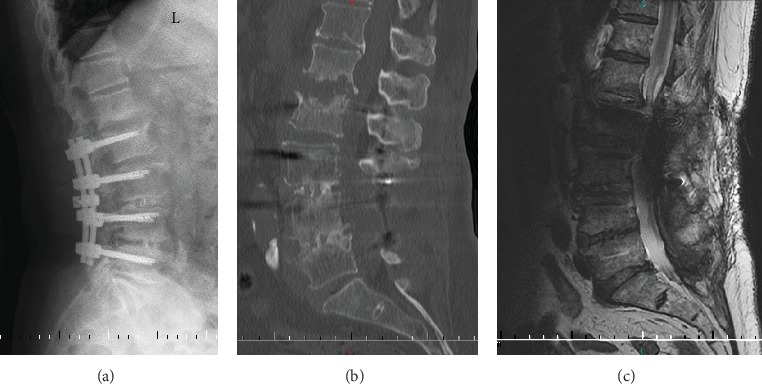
Lateral lumbar radiograph of third hospitalization showing the destructive endplates at the L1-2 space (a). Sagittal CT of third hospitalization showing spondylodiscitis and destruction of bony endplates at the L1-2 segment (b). Sagittal MR T2-weighted imaging of third hospitalization revealing spondylodiscitis at the L1-2 segment (c).
